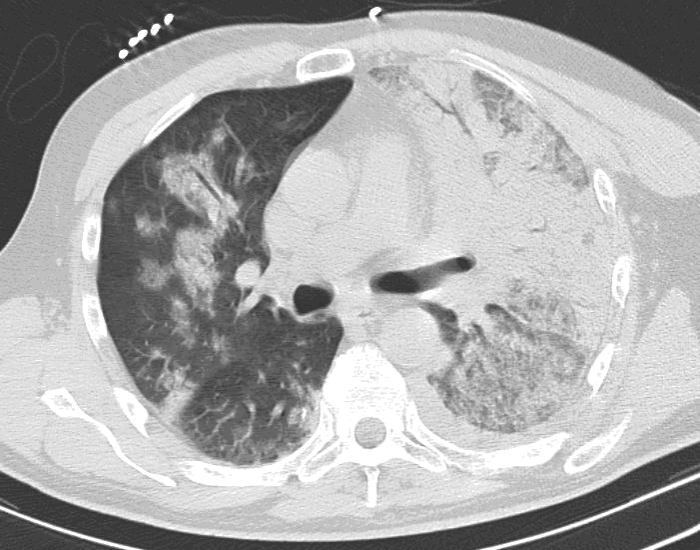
Unenhanced computed tomography scan through the thorax showing alveolar type infiltrates of the two lung field with air bronchograms.
